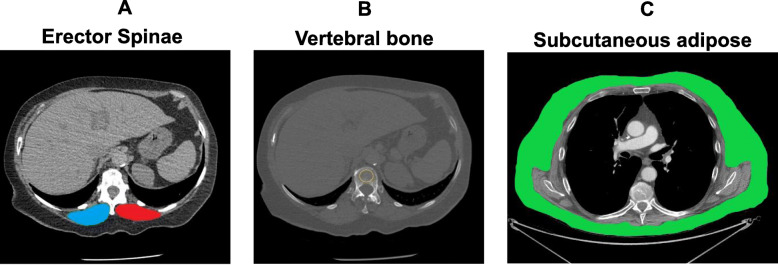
Sample computed tomography (CT) scans used to determine muscle area in our cohort. a Erector spinae muscle cross-sectional area (ESMCSA) measured at the T12 level: blue indicate right muscle and red, left muscle. b Bone density measured in the region of interest (ROI) indicated with yellow circle, at the T12 level. c Subcutaneous adipose tissue (SAT) measured at the T7-8 level, highlighted in green
